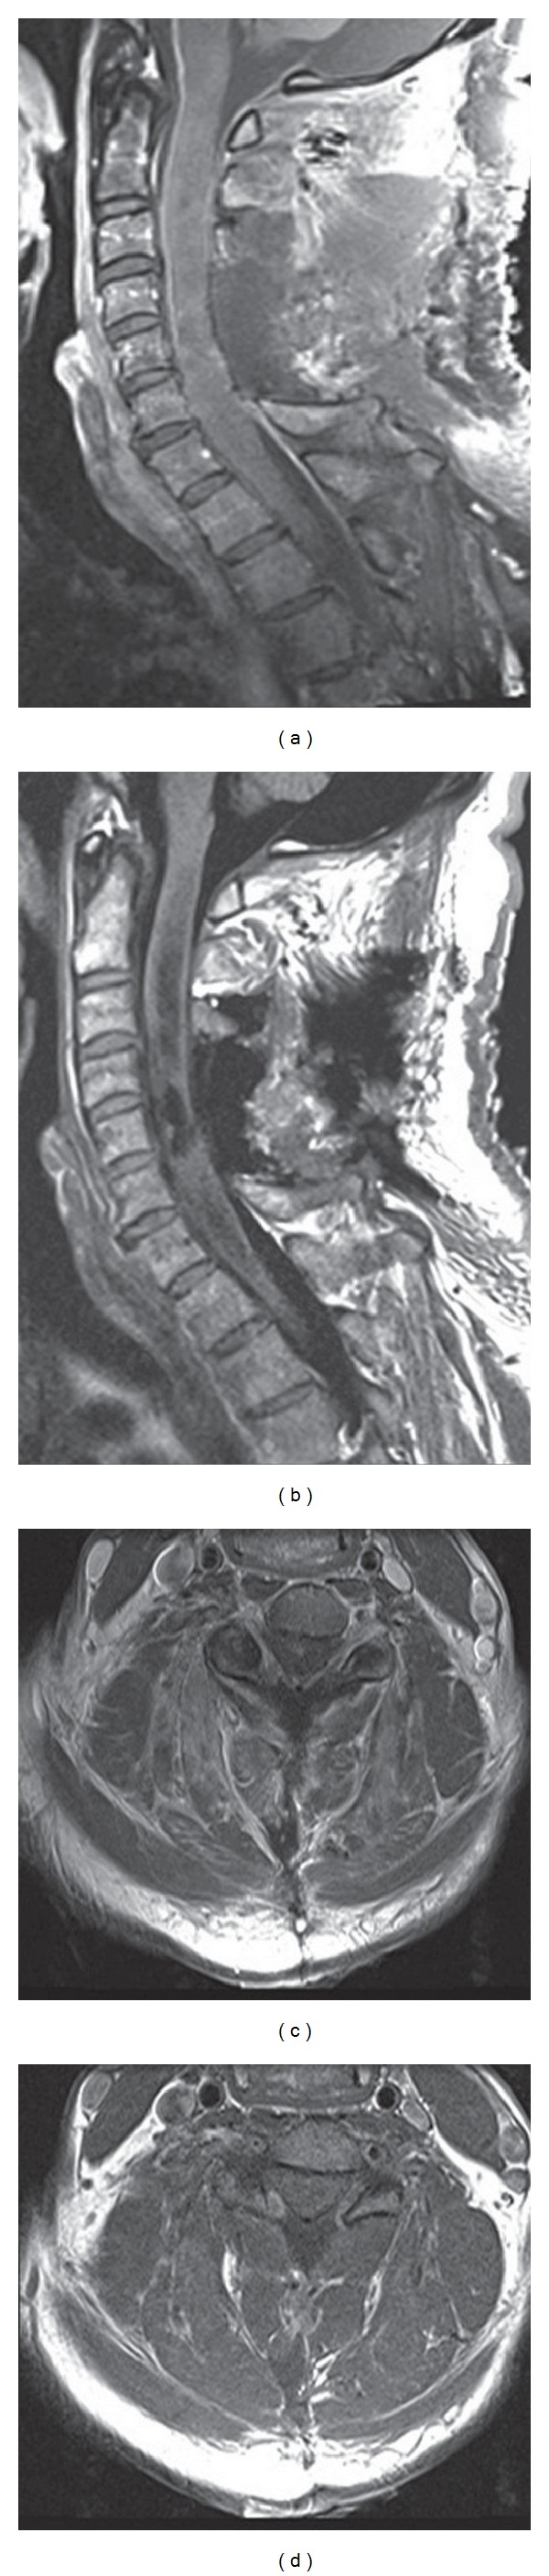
((a), (c)) Sagittal and axial gadolinium enhanced T1-weighted images of the cervical spine after C3–C6 laminectomies and tumor resection demonstrating GTR with no residual enhancement. ((b), (d)) Sagittal and axial T1-weighted inversion recovery images of the cervical spine after tumor resection demonstrating persistent cord syrinx and edema.
